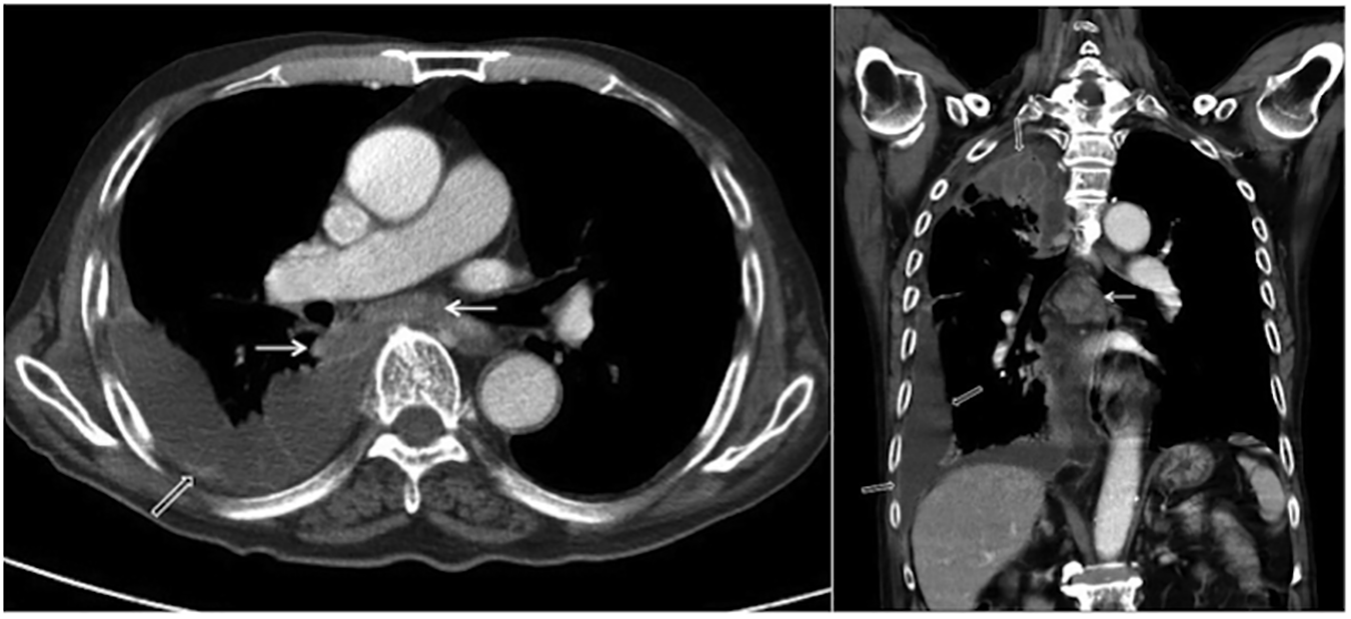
Primary pulmonary tuberculosis pattern with lymphadenopathy and pleural effusion. A 94-year-old male with culture-proven pulmonary tuberculosis and a history of diabetes mellitus. The patient had a glycosylated hemoglobin (HbA1c) of 9.4%. Axial (A) and coronal (B) contrast-enhanced thoracic CT scans revealed loculated right pleural effusion with pleural thickening (open arrows) and enlarged subcarinal and right interlobar lymph nodes with central low attenuation and peripheral rim enhancement (arrows).
